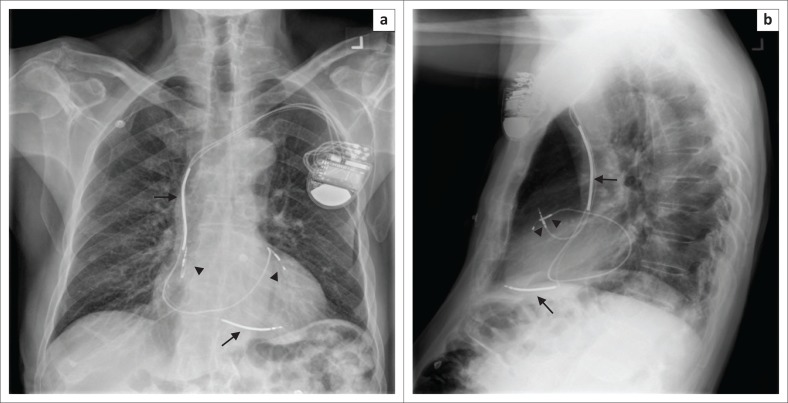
Frontal (a) and lateral (b) chest radiographs showing a cardiac resynchronisation therapy device with its pacemaker leads (arrow heads) in the right atrial appendage and the left ventricle, and its implantable cardioverter defibrillator shock coils (arrows) in the superior vena cava and right ventricular apex.
